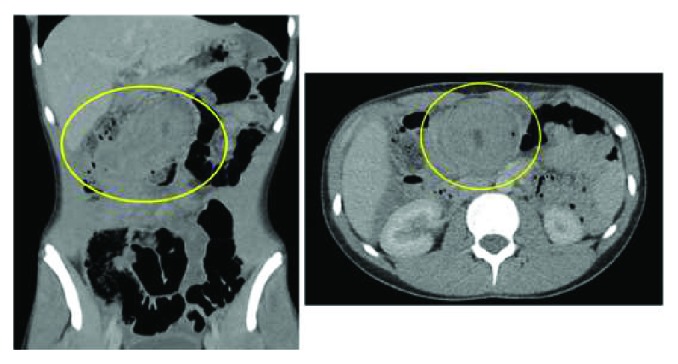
CT imaging indicating intussusception.
